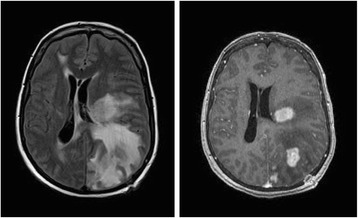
Brain MRI (November 2009): axial FLAIR (left), axial T1 post contrast (right) demonstrating an increase in the size of the left-sided lesions, vasogenic edema, and mass effect leading to subfalcine herniation (notice left parietal burr hole from the biopsy)
